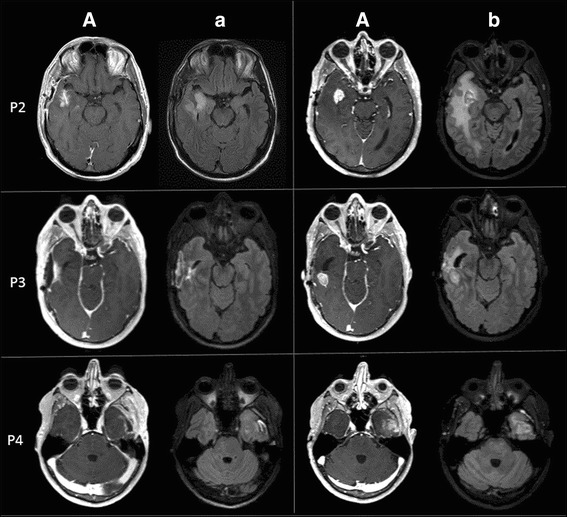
MRI images of the patients P2, P3 and P4 at 4 weeks post-chemoradiation (A and a) and at the time of biopsy or resection (B and b)
